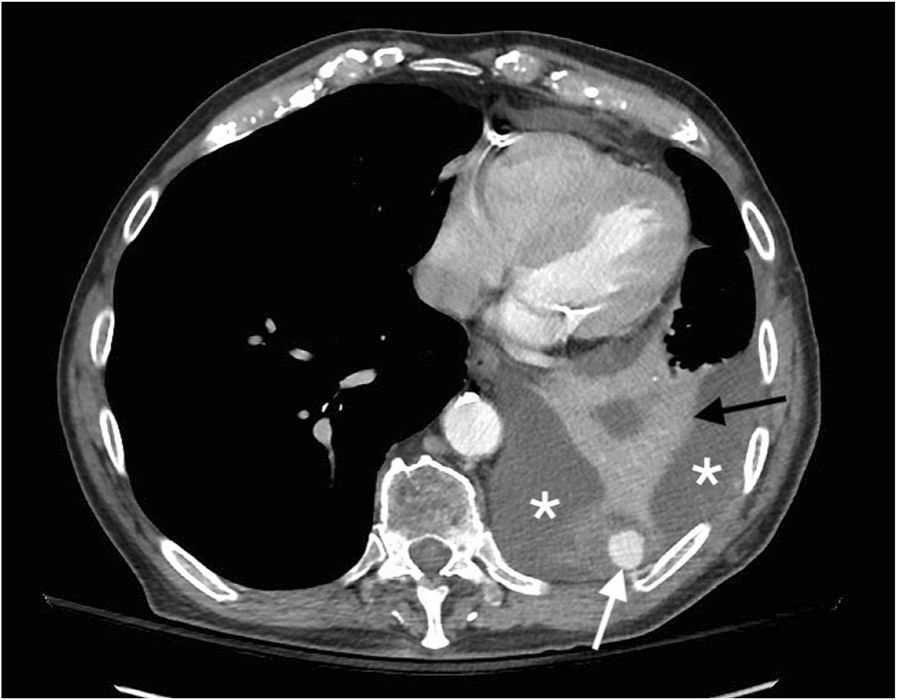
IV contrast-enhanced computed tomography (CT) image, axial projection. The left intercostal artery pseudoaneurysm (white arrow) with hemothorax (asterisks) and adjacent compression atelectasis (black arrow) can be seen
